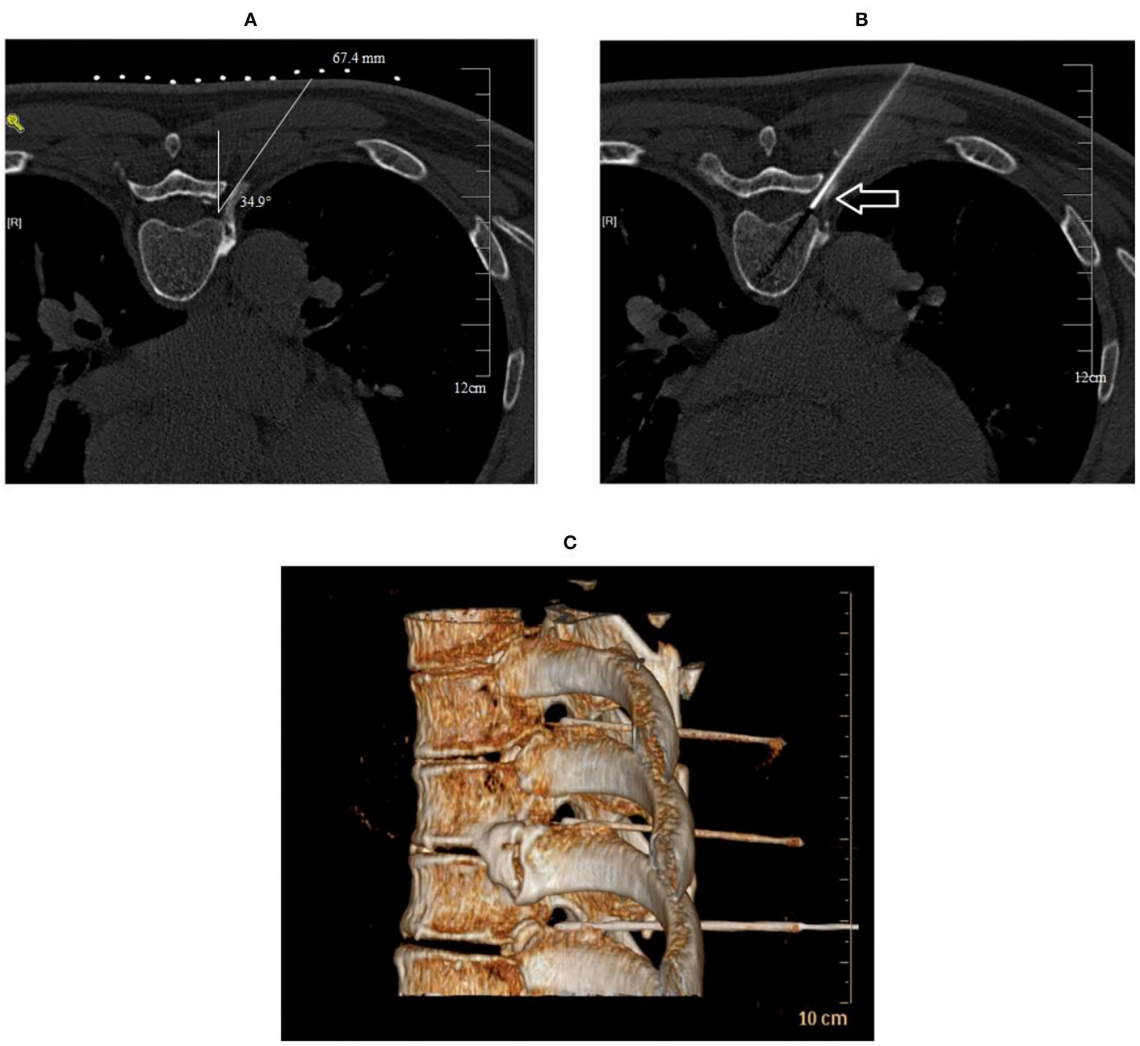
CT-guided puncture route and drug administration. (A) A CT plain scan shows how under CT guidance, a safe route was chosen to avoid injury to the vessel, pleura, and lung. The puncture point and puncture angle were clearly defined by a computer. (B) A CT plain scan shows that the radiofrequency needle was located at the spinal nerve root on the left side and adjacent to the pleura and lung as indicated by the arrow. (C) A 3D CT reconstruction shows the puncture needles of the three segments of the thoracic vertebrae were located at the intervertebral foramen as indicated by the arrow.
